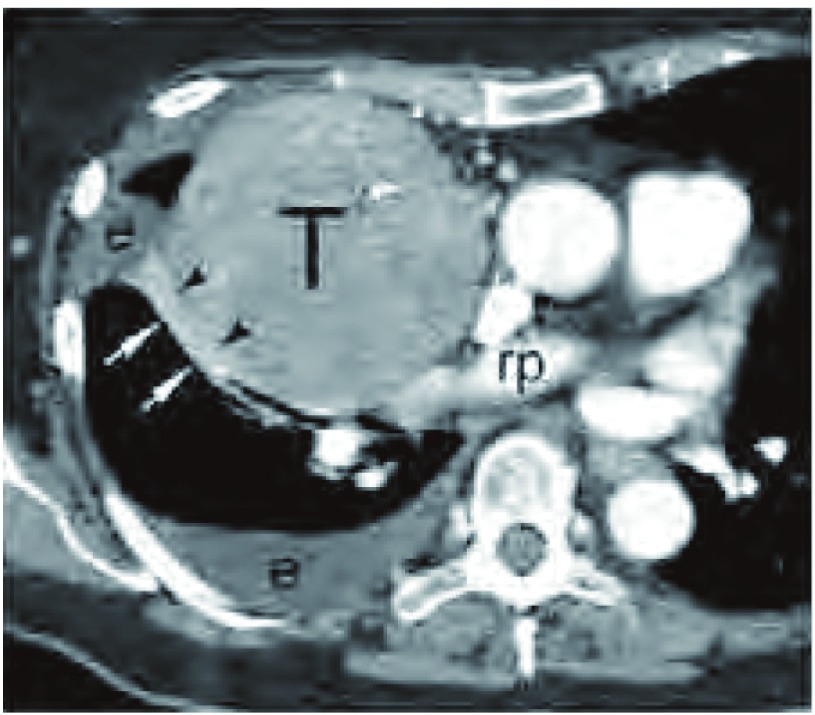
侵犯肺实质。胸部增强CT软组织窗表现，右肺动脉（rp）水平，胸腺瘤压迫右肺中叶支气管，造成中叶肺不张。黑箭头所示不张的肺组织位于肿瘤边缘与右侧叶间裂之间（白箭头所示）。在这个部位很难鉴别肿瘤是否直接侵犯肺实质。注意，有相关的右侧胸腔积液（e）。 Pulmonary parenchymal involvement. Contrast-enhanced chest computed tomography (CT) (soft tissue window), at the level of the right pulmonary artery (rp), shows a thymoma (T) compressing the middle lobe bronchus with resultant atelectasis of the middle lobe. The atelectatic middle lobe is seen between the tumor edge (black arrowheads) and the right major fissure (white arrows). It is difficult to determine whether there is direct tumor invasion into the lung parenchyma at this site. Note the associated right pleural effusion (e).
